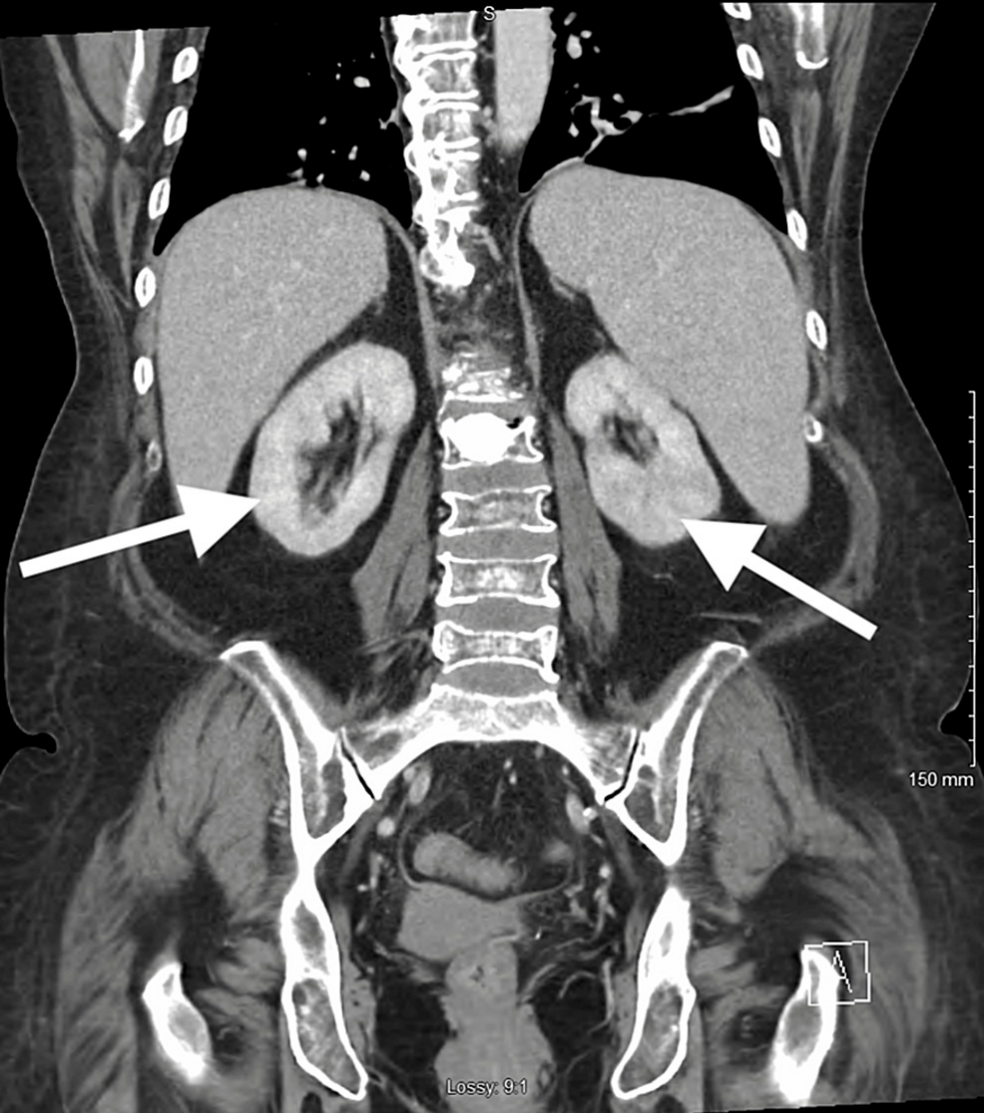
CT Abdomen and pelvis with contrast Findings consistent with evolving bilateral pyelonephritis as noted by white arrows. The left kidney demonstrates a striated appearance suggestive of evolving left pyelonephritis. The right kidney also demonstrates a striated appearance lesser than the left suggestive of evolving pyelonephritis.
